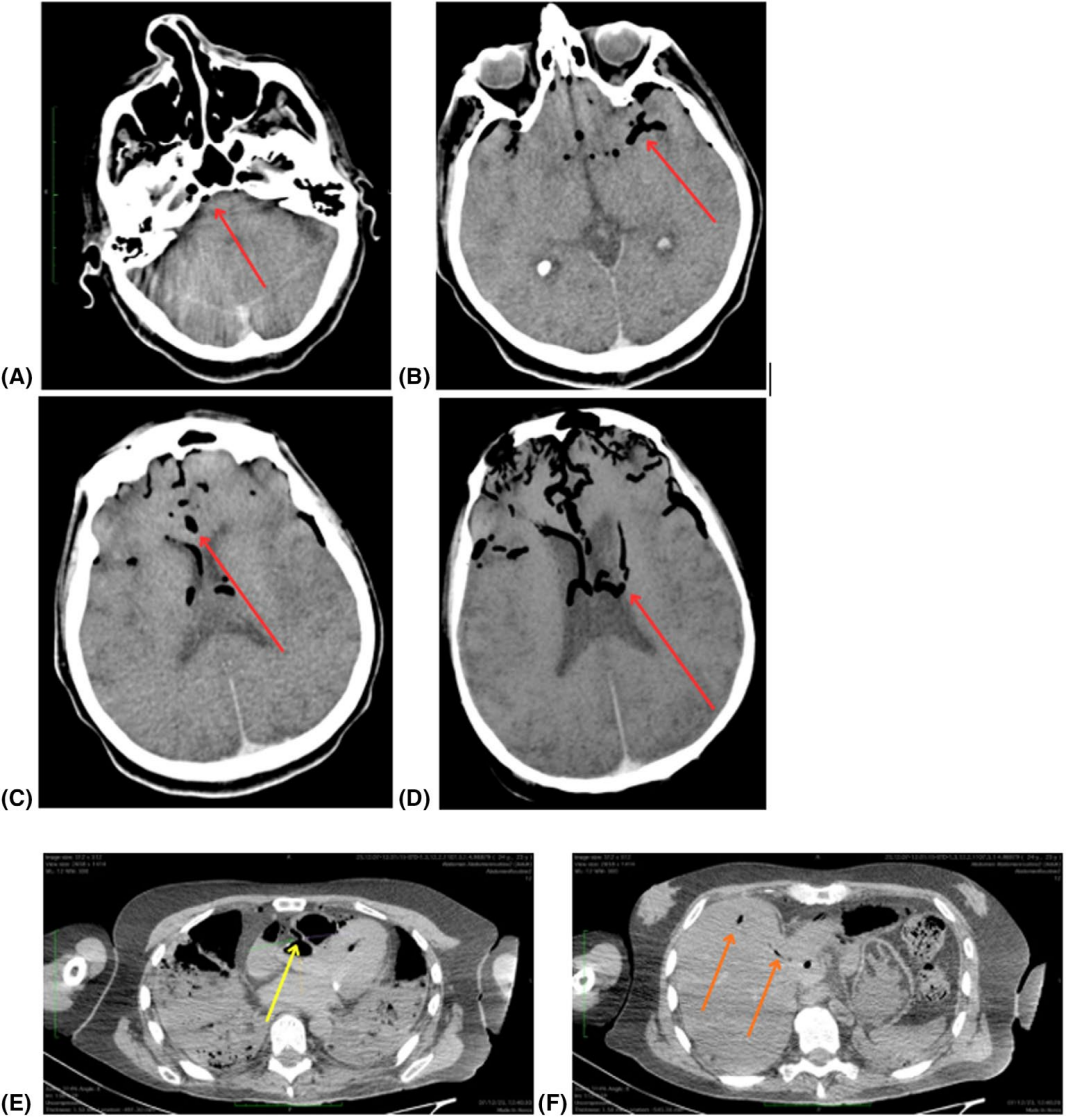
(A–D) Axial brain CT images showing gas within multiple intracranial vessels (red arrows): Basilar artery (A), middle cerebral artery (B), anterior cerebral artery (C), and internal cerebral veins (D). (E) Axial CT image at the level of the ascending aorta and coronary common trunk (yellow arrow). (F) Axial CT image of the upper abdomen showing minimal gas in the left hepatic lobe (orange arrows).
